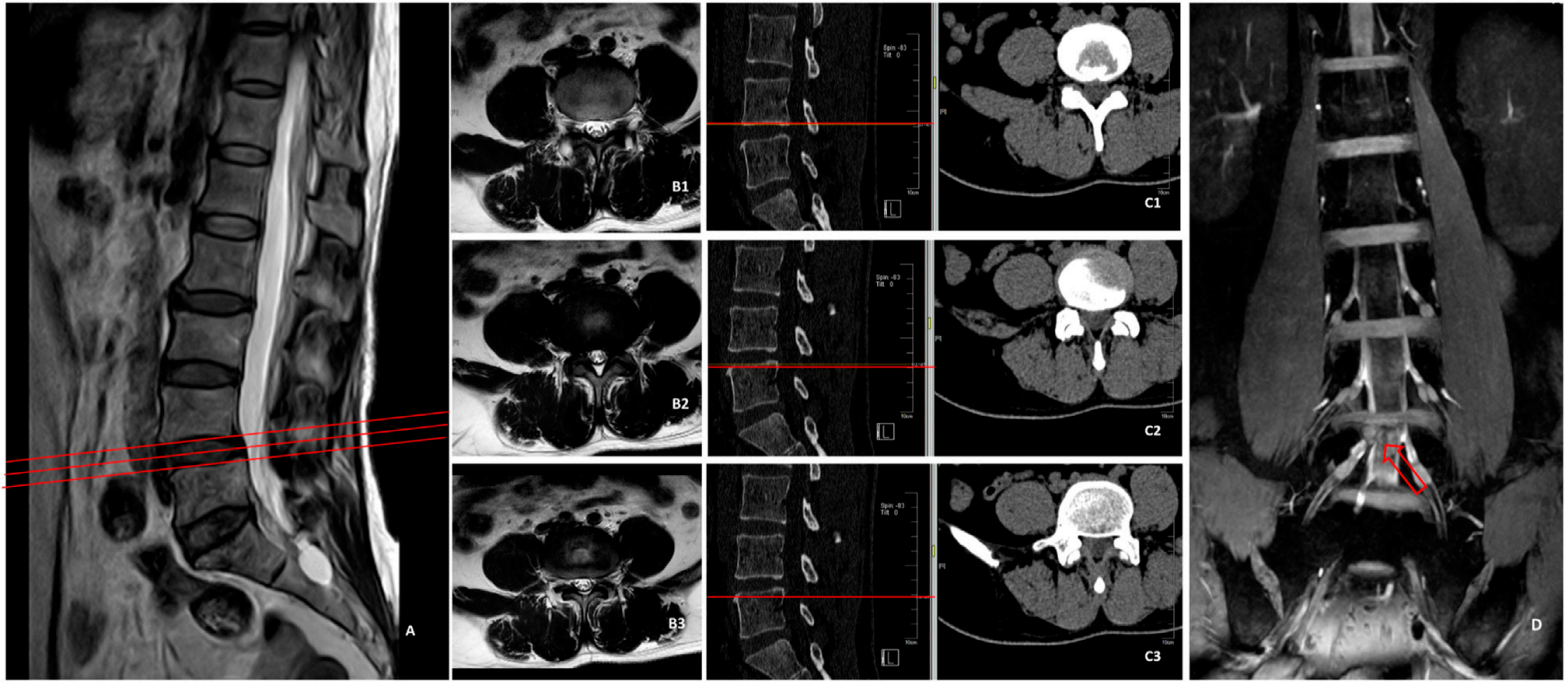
MRI (A,B1–B3) of L4/L5 segment showed no suspicious herniated intervertebral discs, CT (C1–C3) indicated that the right nucleus pulposus was slightly compressed, which was inconsistent with symptoms, while 3D-DESS image (D) indicated that the herniated disc tissue compressed L5 nerve root in the nerve root canal area.
